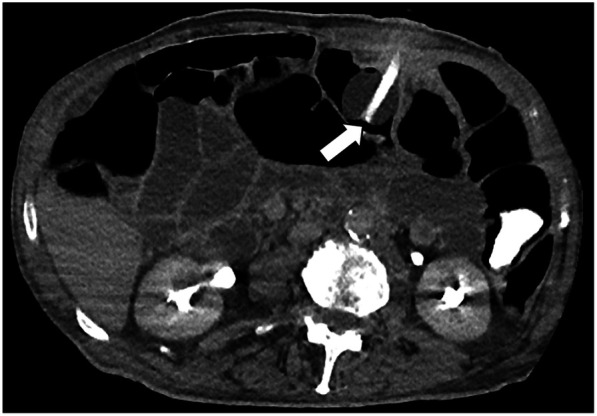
Computed tomography scan revealed the inflated balloon (white arrow) of the percutaneous endoscopic gastrostomy catheter located in the transverse colon after radiological replacement.
